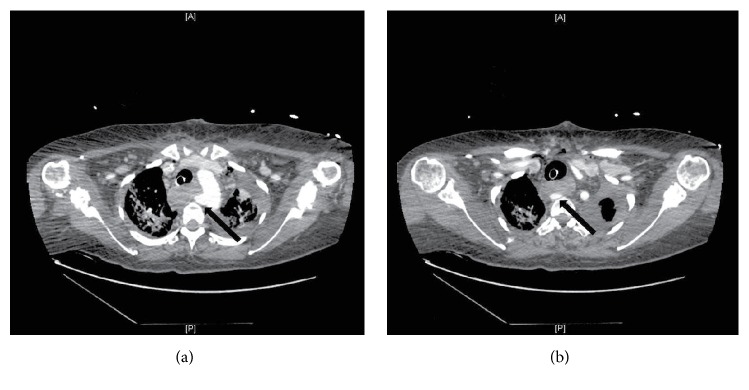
Sequential CT scan images show origin of right subclavian artery from the proximal descending thoracic aorta ((a), black arrow) and coursing posterior to the esophagus ((b), black arrow).
